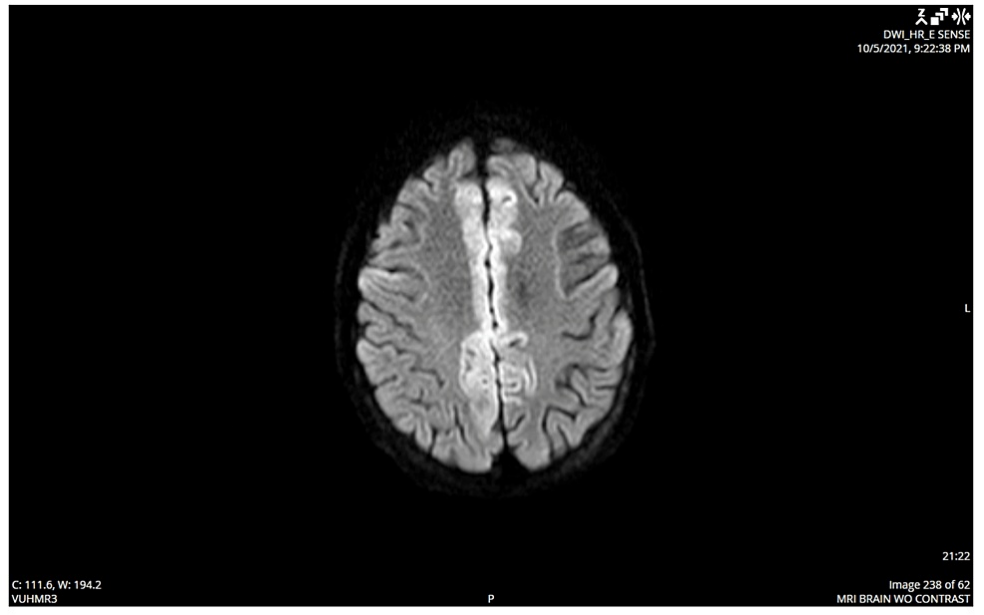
MRI brain without contrast with bilateral parafalcine-restricted diffusion
